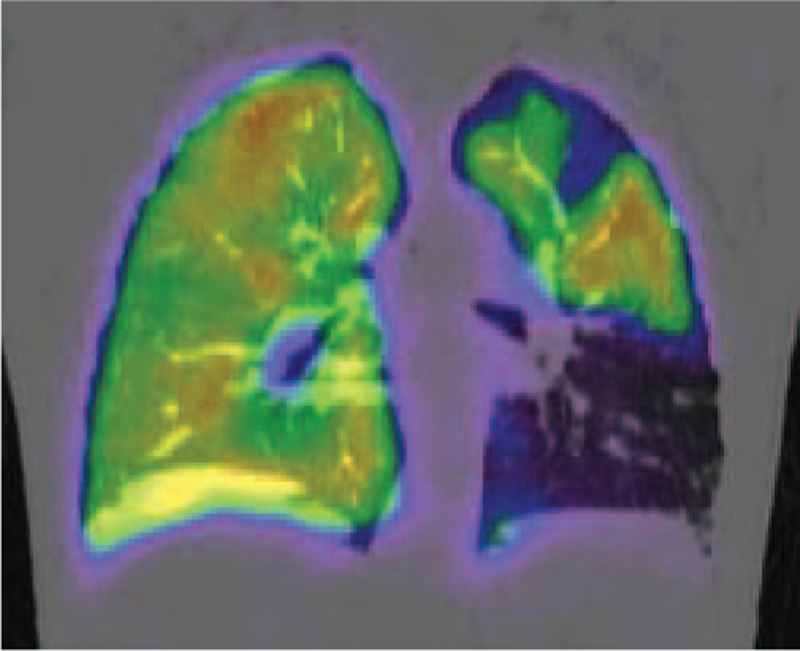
Ventilation perfusion lung scintigraphy showed a mismatch of perfusion and air in the left lung.
